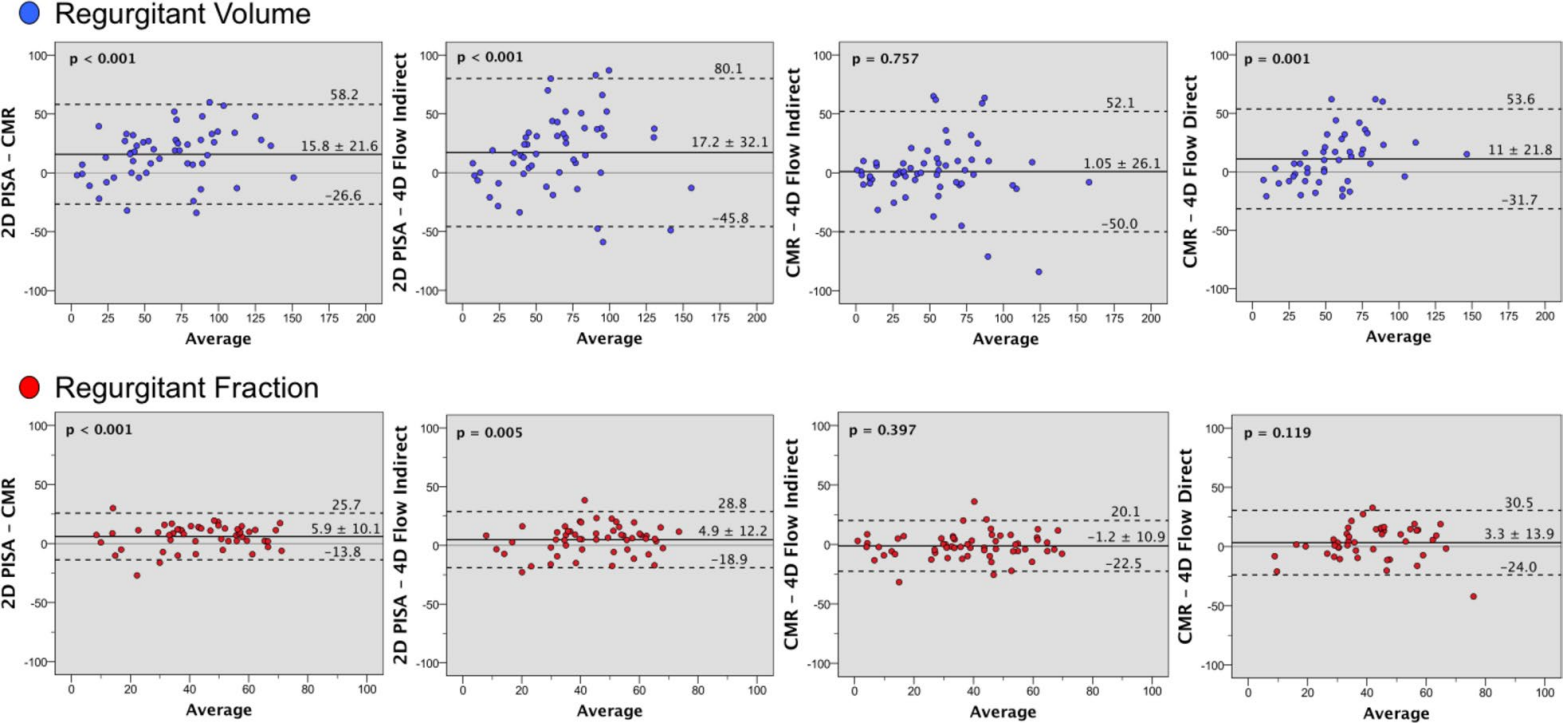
Bland–Altman plots. Agreement of measurements of regurgitant volume (blue dots) and fraction (red dots) by 2D-echocardiographic flow convergence method (2D-PISA), standard cardiac magnetic resonance (CMR), and 4D flow CMR indirect and direct methods, in patients with mitral valve prolapse
